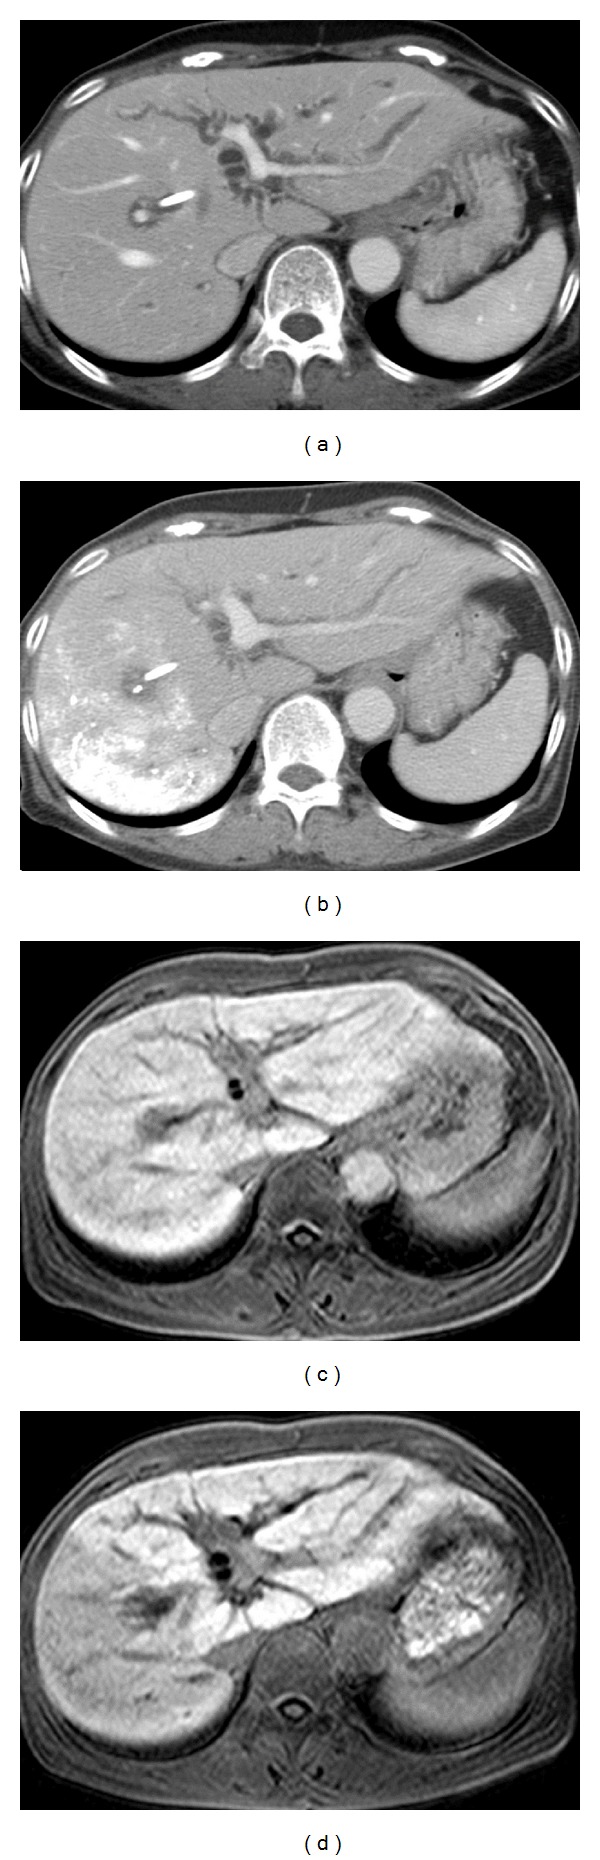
CT and MRI findings before and after PVE. (a) Contrast-enhanced CT before PVE showing a homogenous enhancement effect in the whole liver parenchyma. (b) CT 3 weeks after PVE of the right portal vein, showing accumulation of iodized oil mixed with ethanol in the right hepatic lobe and hypertrophy of the left lobe. (c) Gd-EOB-DTPA-enhanced MRI before PVE, also showing a homogenous enhancement effect. (d) Gd-EOB-DTPA-MRI 1 week after PVE of the right portal vein, showing a reduction in SI in the right hepatic lobe.
